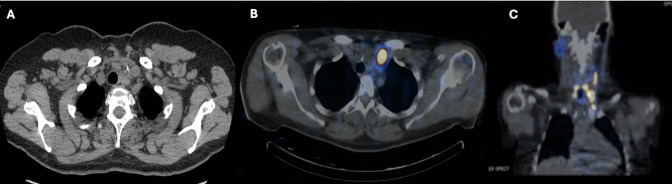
Post-thyroidectomy nuclear medicine I-123 whole-body single-photon emission computed tomography (SPECT) (A) axial view; (B), coronal view) showing marked radiotracer uptake in the left neck extending leftward and superiorly to the level of the thyroid cartilage. Post-thyroidectomy neck CT without contrast (C) showed asymmetrical enlargement of the left internal jugular vein.
